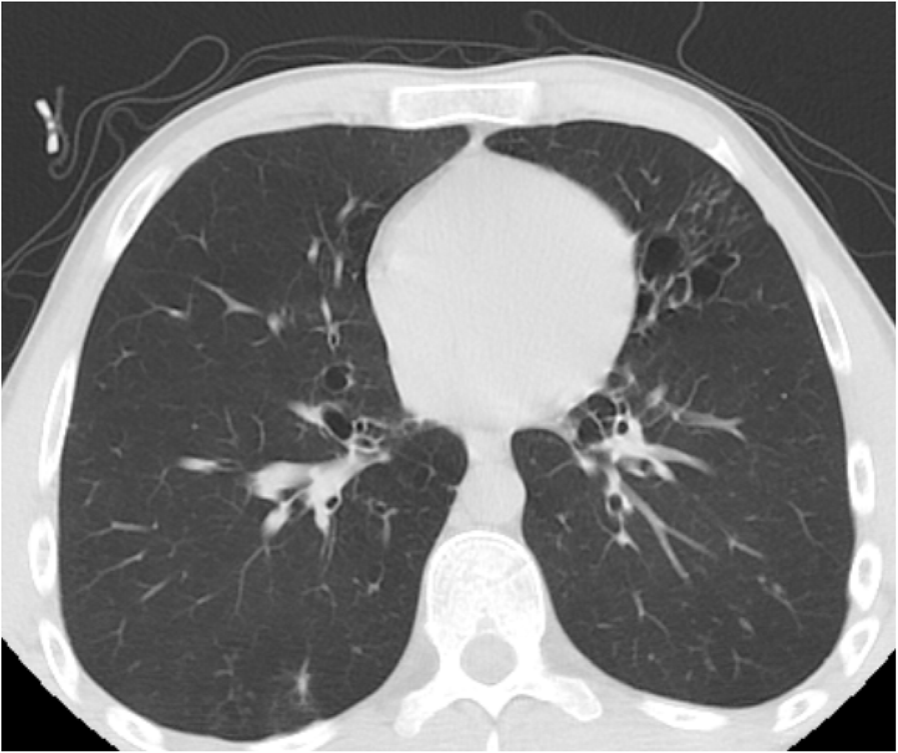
No typical signs of COVID-19The CT-image shows bronchiectasis, bronchial wall thickening—CO-RADS 2. There are no ground glass opacities
